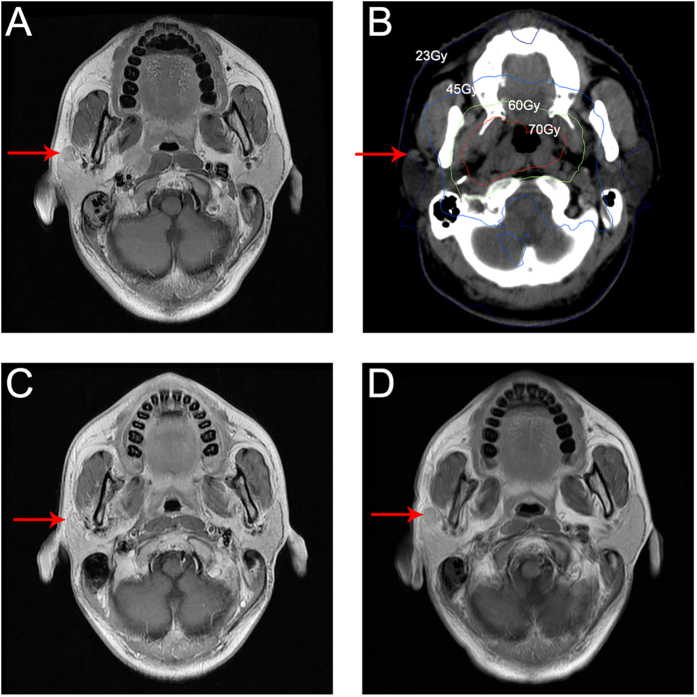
Imaging of parotid lymph node involvement in the right parotid gland in a 29-year-old male with NPC. (A) Pretreatment transverse T1-weighted spin-echo MRI image showing a 10 mm nonspecific hypointense nodule within the right parotid gland. (B) Isodose curves for intensity-modulated radiotherapy: red, 70 Gy; green, 60 Gy; light blue, 45 Gy; blue 23 Gy. (C) Transverse T1-weighted spin-echo MRI image 3 months after radiotherapy showing the lesion within the right parotid gland was controlled. (D) Transverse T1-weighted spin-echo MRI image 32 months after radiotherapy showing a 12 mm nonspecific hypointense nodule within the right parotid gland, which was pathologically confirmed as squamous carcinoma after surgery.
